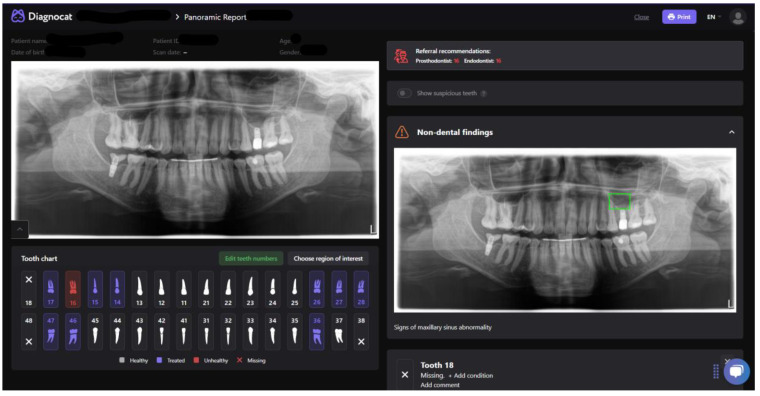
Screenshot of the AI Program “Diagnocat” (DGNCT LLC, Miami, FL, USA)—Report from Panoramic Radiograph Analysis.
